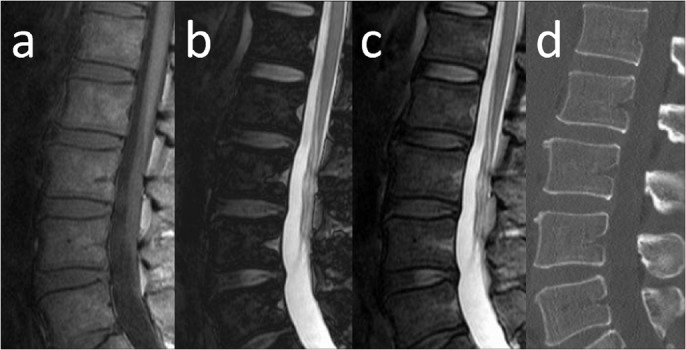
MRI of the lumbar spine in a false-positive case misclassified on the basis of β_2_-microglobulin. Sagittal MR images and sagittal reconstructed CT of a 53-year-old man with asymptomatic myeloma and a fat-signal fraction of 64% are shown. Serum creatinine level was 2.4 mg/dl, β_2_-microglobulin was 5.99 mg/l, and BMPC% from the iliac crest was 7.5%. The elevated β_2_-microglobulin level might have resulted from renal impairment. a) T1-weighted imaging shows diffusely decreased signal intensity in bone marrow. Bone marrow signal intensity is almost equal to that of intervertebral disc. b) Calculated out-phase image. c) Calculated in-phase image. d) Sagittal reconstructed CT.
